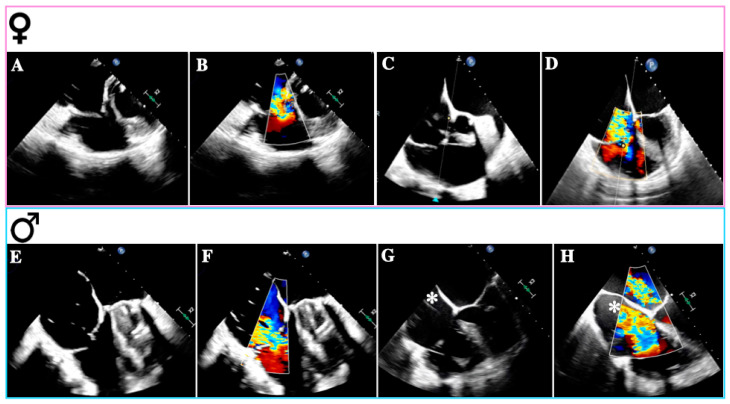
Causes of tricuspid regurgitation according to sex. Transesophageal echocardiogram—mid-esophageal views: (A): Rheumatic tricuspid regurgitation. (B): Rheumatic tricuspid regurgitation with color Doppler. (C): Atrial secondary tricuspid regurgitation. (D): Atrial secondary tricuspid regurgitation with color Doppler. (E): Ventricular secondary tricuspid regurgitation. (F): Ventricular secondary tricuspid regurgitation with color Doppler. (G): Lead-related tricuspid regurgitation. (H): Lead-related tricuspid regurgitation with color Doppler. The images (A–D) were obtained from female patients; (E–H) were obtained from male patients; * denotes the device-lead.
